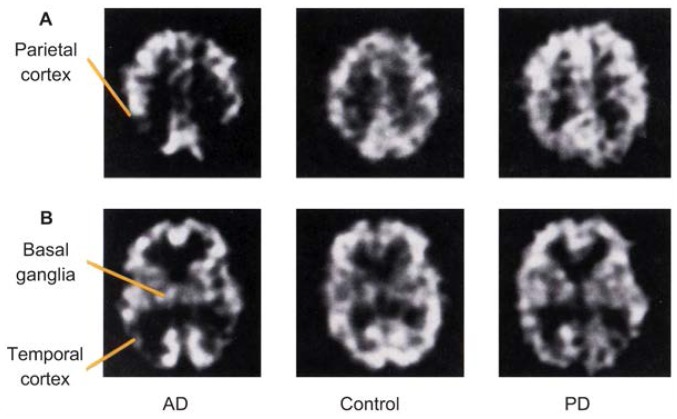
Single-photon emission computed tomography (SPECT) images representing two horizontal cross-sections through the brains of an Alzheimer’s disease (AD) patient, a control subject, and a Parkinson’s disease (PD) patient. The images in row A include the parietal cortex; the images in row B include the basal ganglia and the temporal cortex. Compared with the control subject, the AD patient exhibits strongly reduced blood flow in the parietal and temporal cortices. In the PD patient, blood flow in the pari­etal and temporal cortices is reduced compared with that in the control subject but not as much as in the AD patient.
